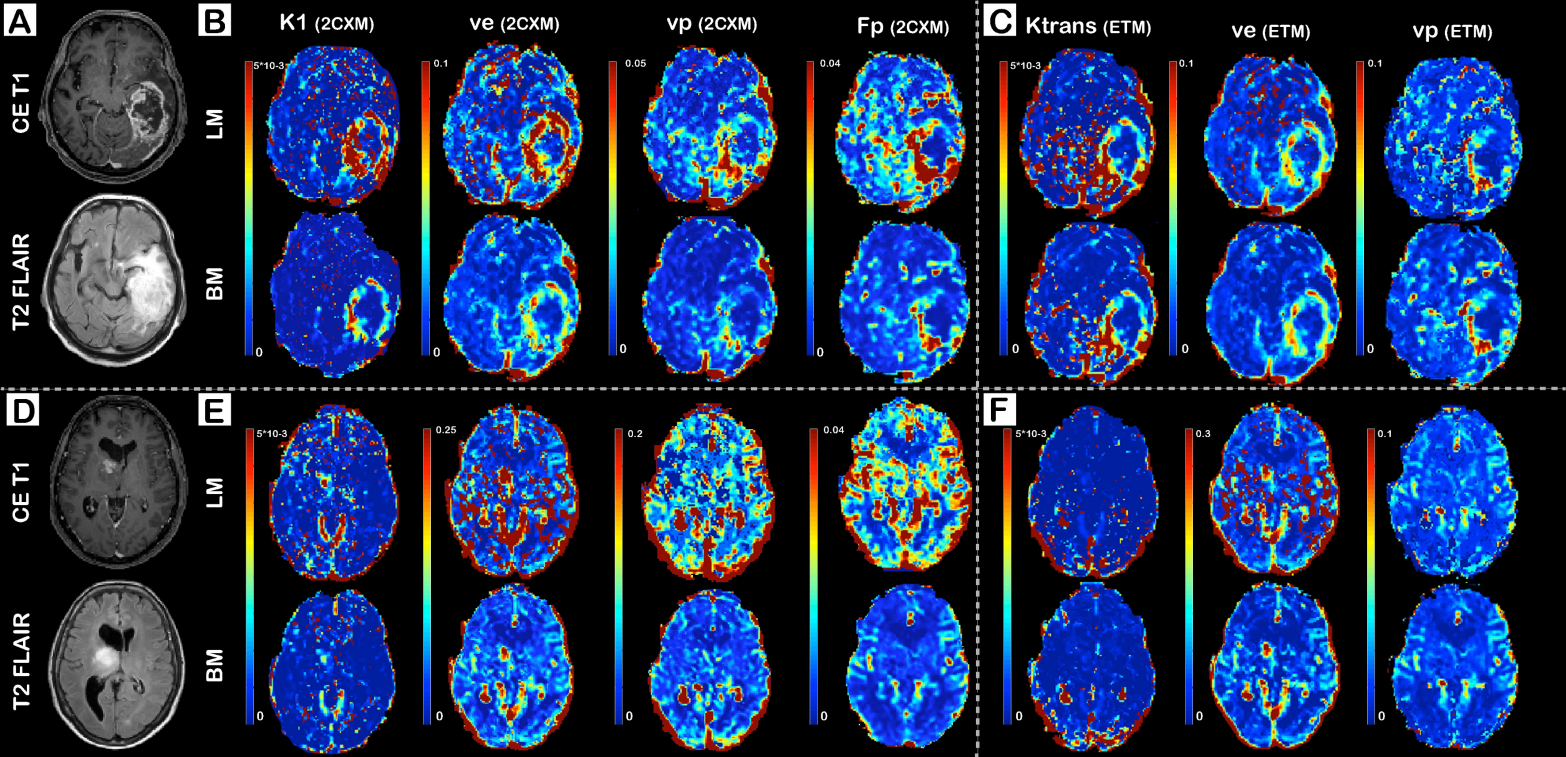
Representative K_1_ (permeability rate), K_trans_ (leakage-flow), V_e_ (interstitial volume), v_p_ (plasma volume), and F_p_ (plasma flow) images of a patient with glioblastoma multiforme (A-C) and astrocytoma grade II (D-F). Dynamic Contrast Enhanced MRI data are fitted to the 2-compartment-exchange model (2CXM; B and E) and the extended Toft’s model (ETM; C and F) using either the Levenberg-Marquardt (LM) method or Bayesian modeling (BM). Conventional images (contrast enhanced T1, CE T1, and T2 FLAIR) are demonstrated in (A) and (D).
